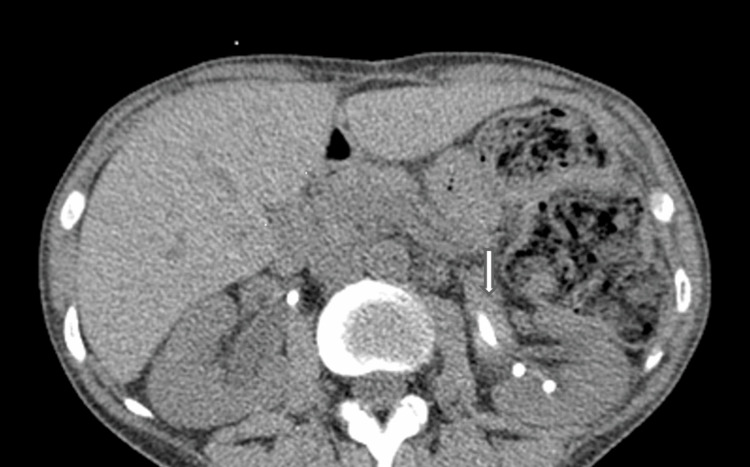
Plain CT showing increased density within a dilated left renal tract (white arrow) indicating distension with blood/acute thrombus.
